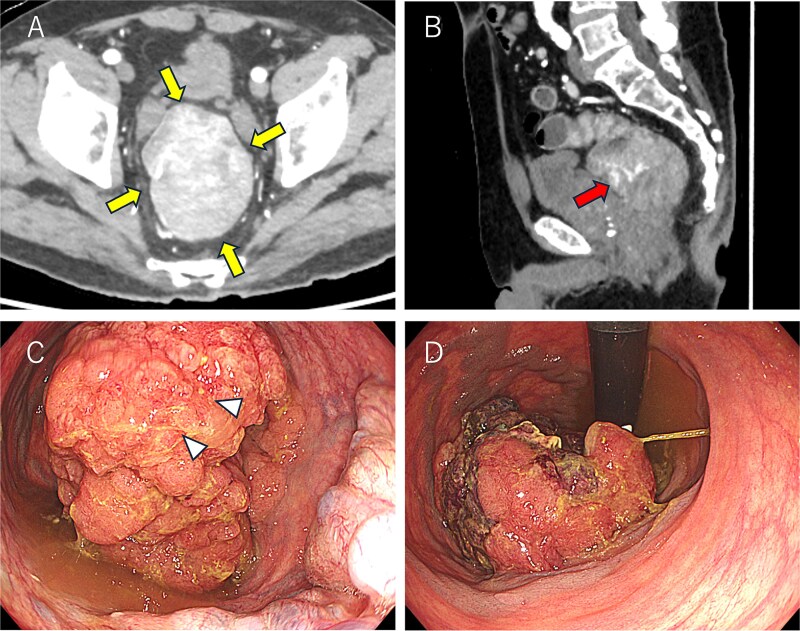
Colonoscopy and computed tomography findings. (A) A mass measuring 8.5 × 6.5 cm was observed in the lower rectum (arrow). (B) A vascular stalk-like structure was noted in the proximal portion of the tumor (arrow). (C, D) A large mass occupying the rectal lumen was observed 3 cm from the anal verge (D: retroflexed endoscopic view). The arrowhead indicates the biopsy site.
